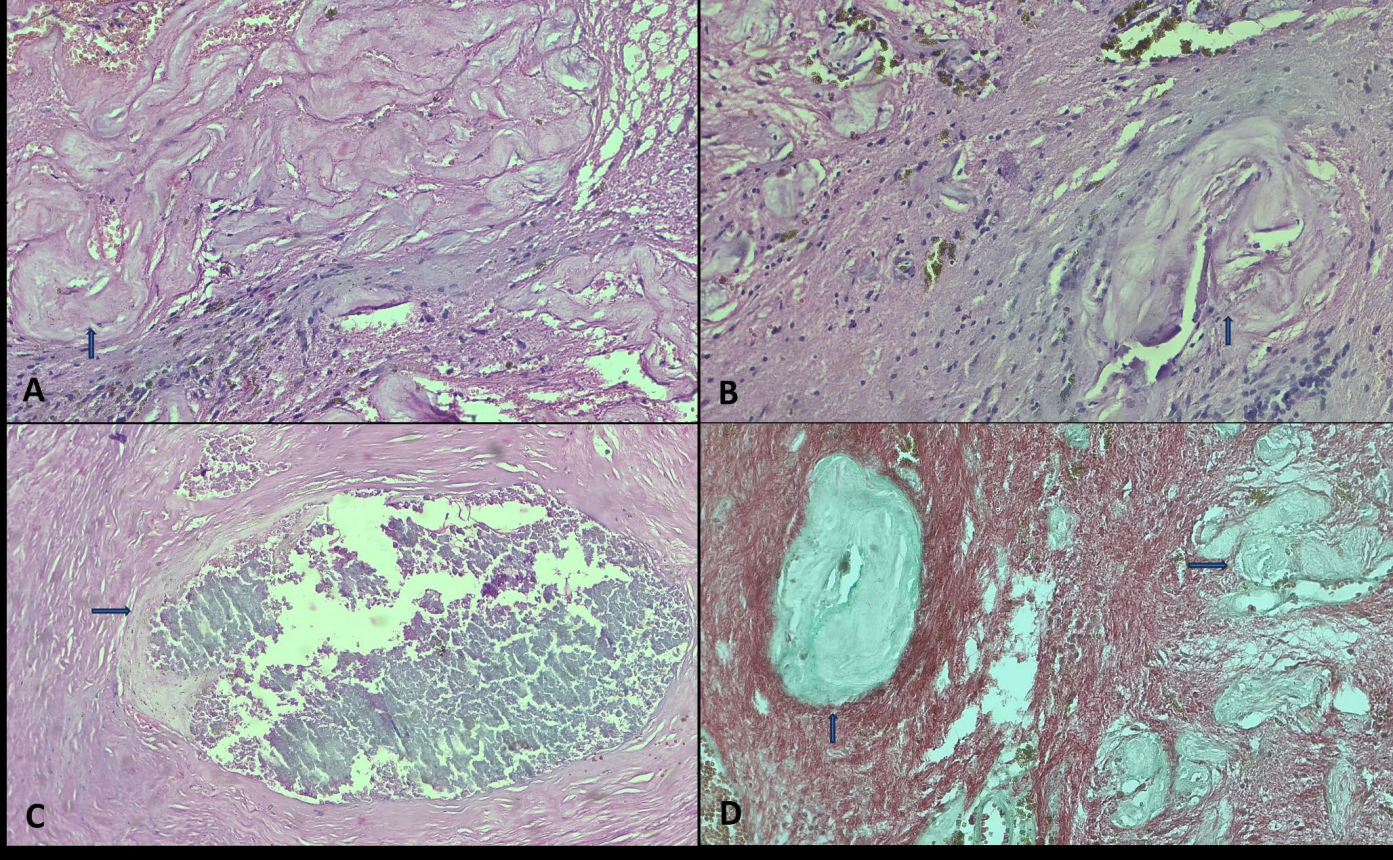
A: A 44-year-old male patient underwent GKRS (13 Gy) due to a hemorrhagic CM in the right mesencephalon. B: The patient’s diffusion MRI at the fourteenth month showed edema at the level of the crus cerebri, and the patient’s complaint of diplopia became permanent. C: A 24-year-old male patient underwent GKRS (15 Gy) due to a CM at the left centrum semiovale. D: The patient’s computed tomography at six months revealed perilesional edema. The dysesthesia in the patient’s right arm became permanent during follow-ups
